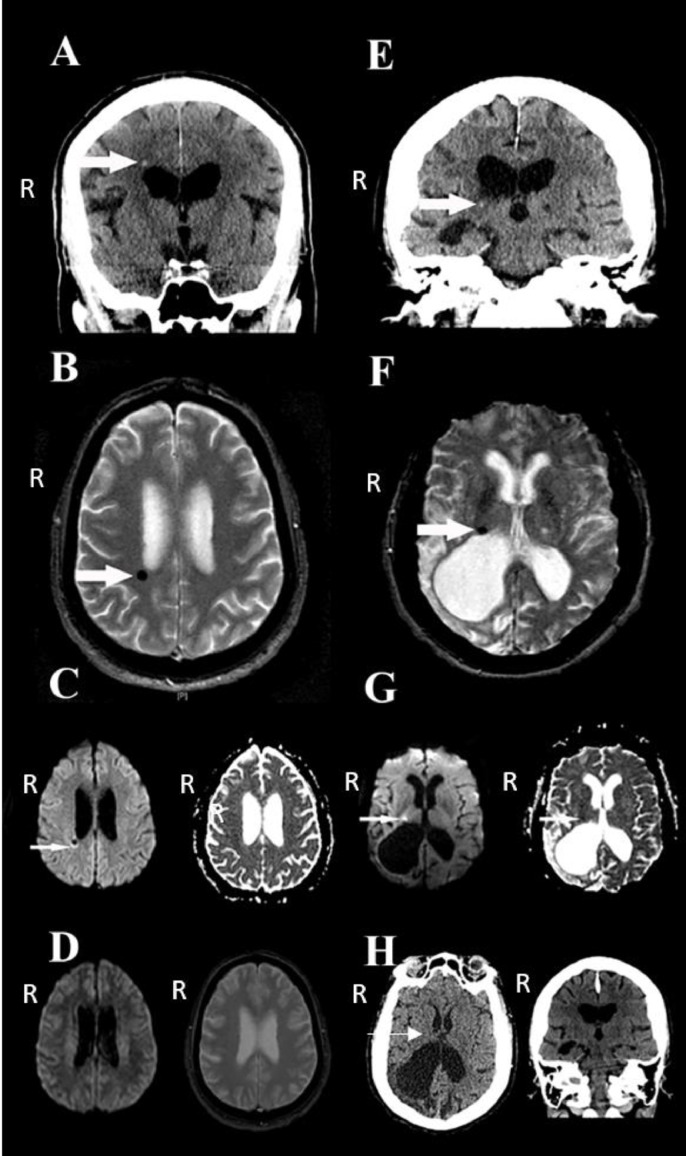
Case 1 images are in the left column (panels A–D) and case 2 in the right column (panels E–H). A and E. Coronal reconstructions using high resolution (1.25 mm slice thickness) unenhanced head CT demonstrates areas of small acute hemorrhage (arrow). B and F. Axial T2*GRE image demonstrating cerebral microbleeds (arrows). C and G. Axial diffusion weighted image (DWI) in the left and apparent diffusion coefficient (ADC) in the right for each case demonstrating hypointense lesion surrounded by hyperintense rim in case 1 (arrow) and hyperintense DWI signal with corresponding hypointense ADC signal for case 2 (arrows). D. DWI (left) and T2*GRE (right) images predating the occurrence of CMB show absence of the lesions for case 1. H. Axial and coronal high resolution (1.25 mm slice thickness) unenhanced head CT predating the occurrence of CMB in case 2 demonstrating absence of the acute hemorrhage (arrow).
